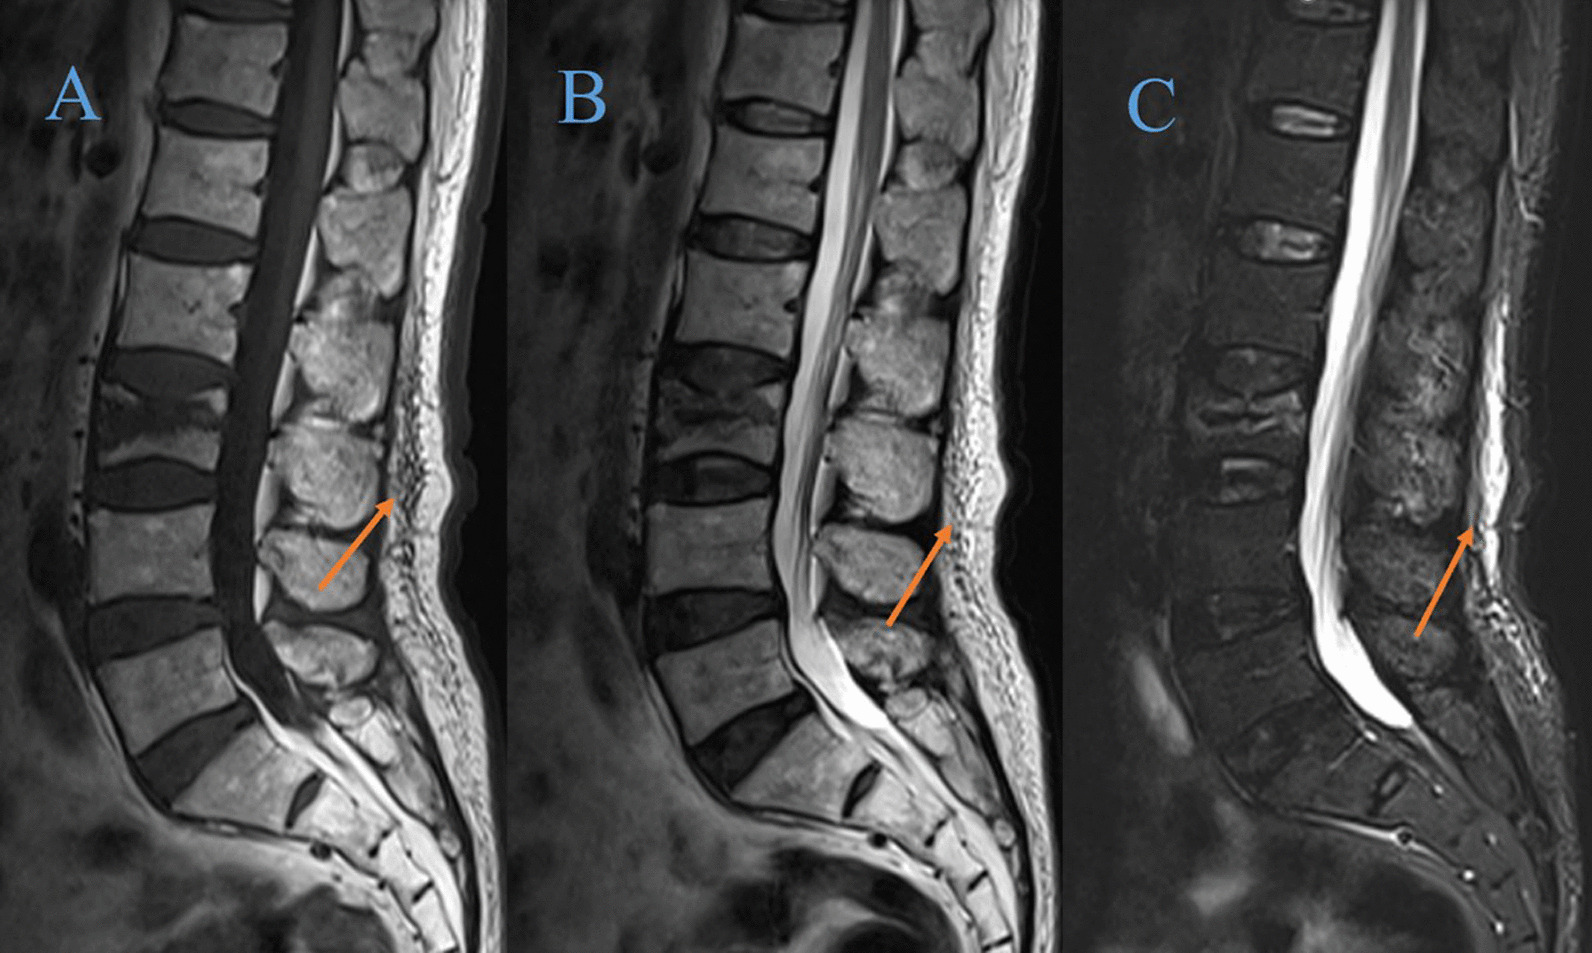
Posterior fascia oedema was visible on T1-WI (A, orange arrow), T2-WI (B, orange arrow) or STIR images (C, orange arrow) 0f MRI
